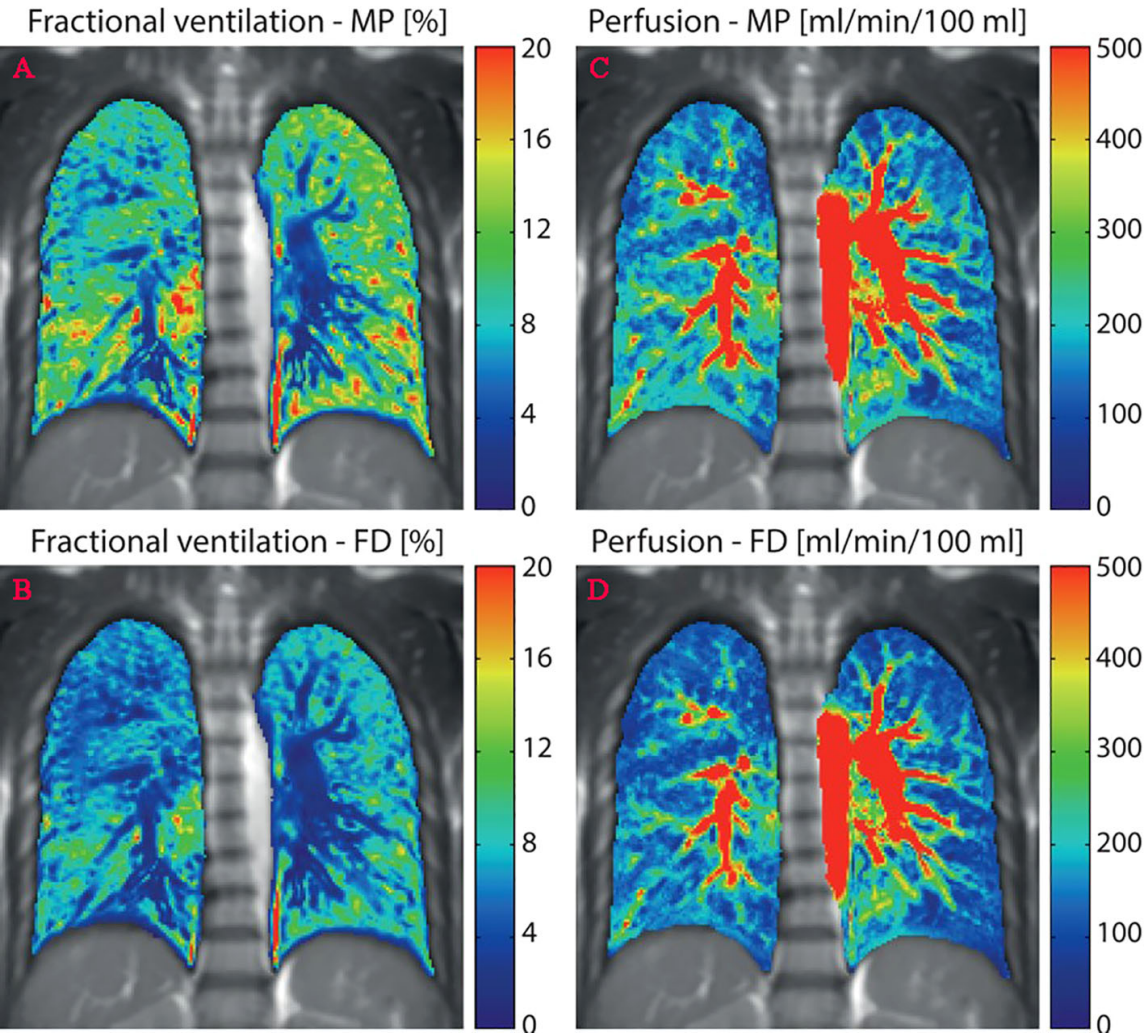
Quantitative maps of ventilation and perfusion calculated using the matrix pencil (MP) and Fourier decomposition (FD) methods. (A, C), MP-MRI ventilation/perfusion; (B, D), FD-MRI ventilation/perfusion. Reproduced from reference (64) with permission from Wiley, copyright 2016.
